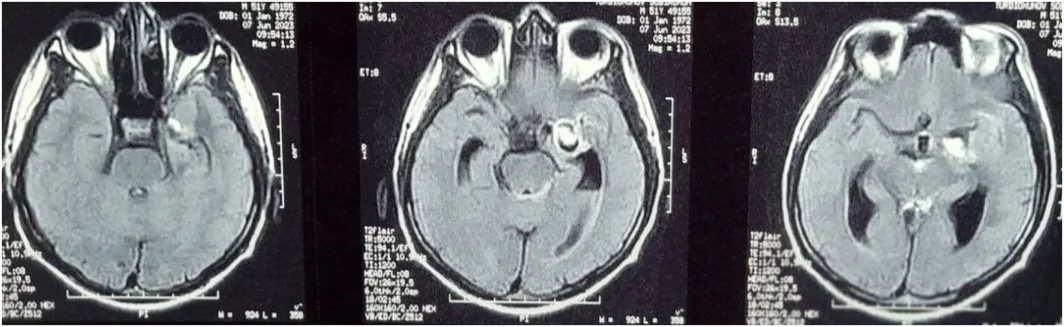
Brain MRI demonstrating a giant left MCA aneurysm. The aneurysm shows variable signal intensity, including T1 hyperintensity (first image), T2 hypointensity (middle), and heterogeneous signal on T2‐FLAIR (last), suggesting thrombosis of most of the aneurysm sac. The peripheral T1 hyperintensity corresponds to laminated mural thrombus of differing ages, whereas the small residual patent lumen appears as a central flow void. The T2 hypointensity reflects the organized thrombus and the heterogeneous T2‐FLAIR signal reflects the mixed composition of the sac. The lesion arises from the left middle cerebral artery and produces local mass effect on the adjacent parenchyma without surrounding vasogenic edema.
